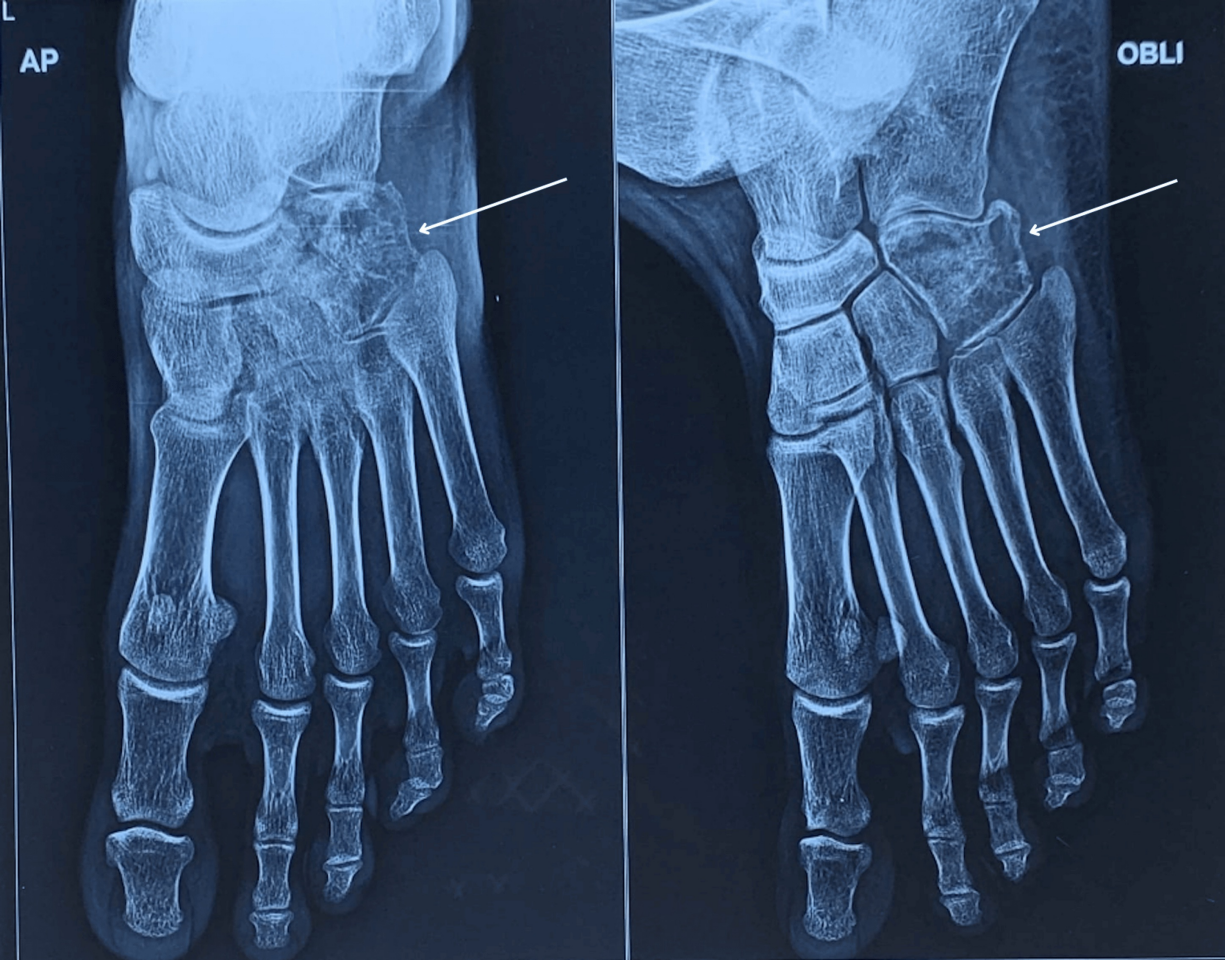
Radiograph of left foot (anteroposterior and oblique views) on presentation showing osteolytic lesion of cuboid.
